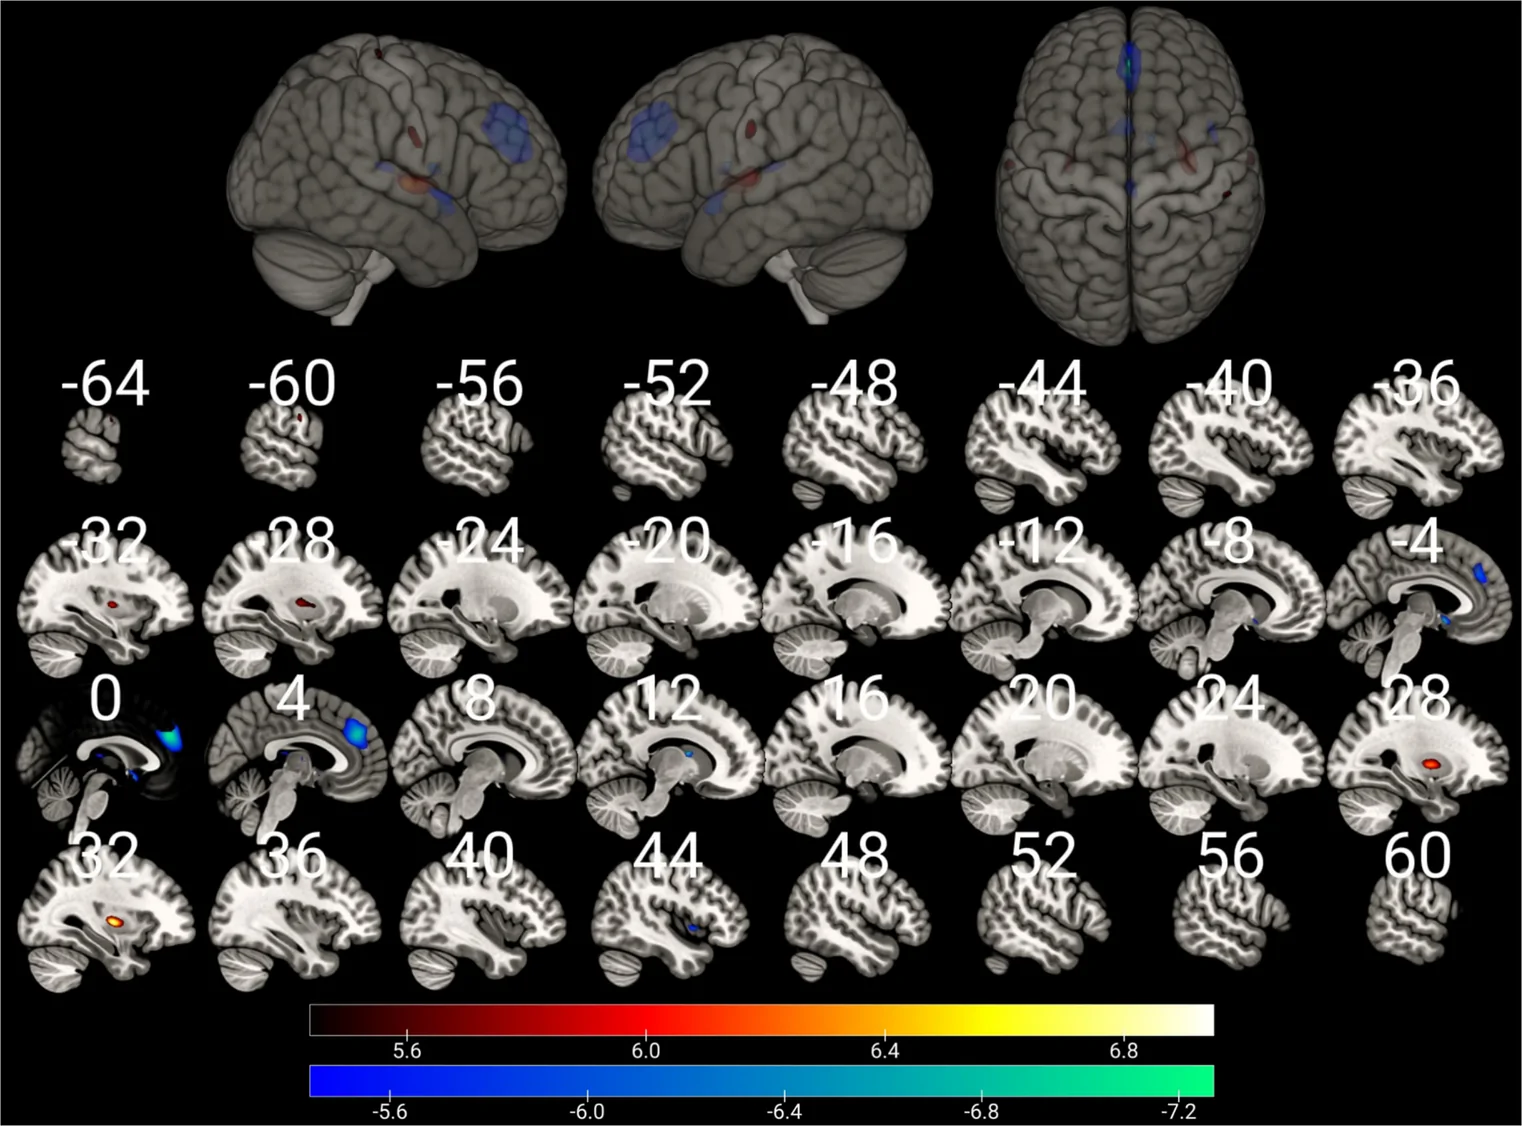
Regional [^18^F]FDG PET alterations in glucose metabolism in patients with DLB (ADNI cohort) when compared to healthy controls, corrected for disease stage at autopsy. Hypermetabolism in non-cortical regions is observed in the bilateral putamen. Subcortical hypometabolism is present in the bilateral splenium of corpus callosum; corpus of right caudate nucleus; right medial thalamic complex. Regarding cortical regions, hypermetabolism is seen in the hand area of the bilateral postcentral gyrus. Hypometabolism is seen in the right insula; left olfactory cortex; bilateral superior frontal gyrus; bilateral anterior cingulate cortex. Regions of significant differences in hypometabolism and hypermetabolism are displayed on blue–green and red–white scales, respectively, with darker blue indicating greater hypometabolism and brighter white indicating greater hypermetabolism. DLB = Dementia with Lewy bodies; FDG = [^18^F]Fluorodeoxyglucose
